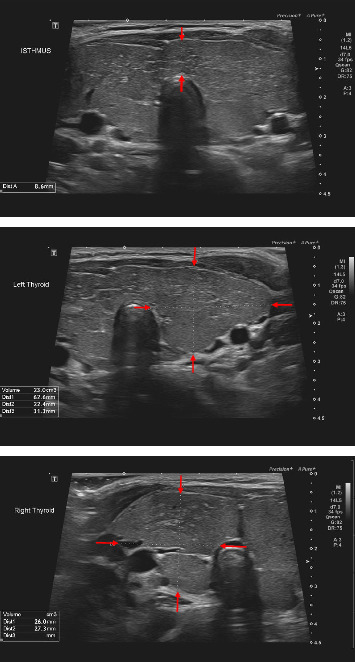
Transverse view of thyroid ultrasonography scan shows enlarged bilateral thyroid and isthmus with heterogeneous parenchyma and increased Doppler color flow. No discrete nodules or regional lymphadenopathy were noted. Dimensions for the right lobe, left lobe, and isthmus were (2.6 × 2.7 × 7.4 cm), (2.2 × 3.1 × 6.3 cm), and (8.6 mm for anteroposterior depth), respectively.
